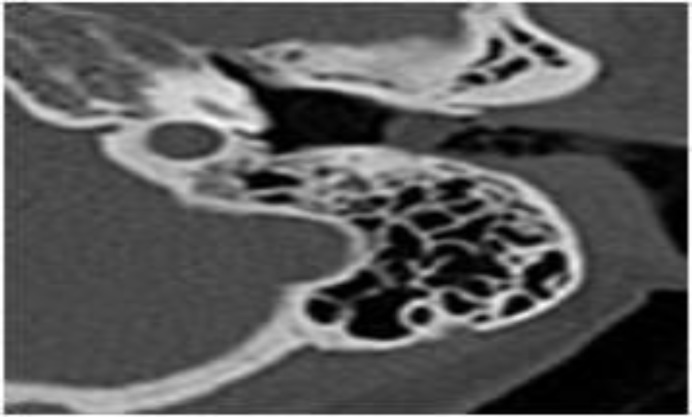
CT scan showing narrowing of the left external auditory canal
